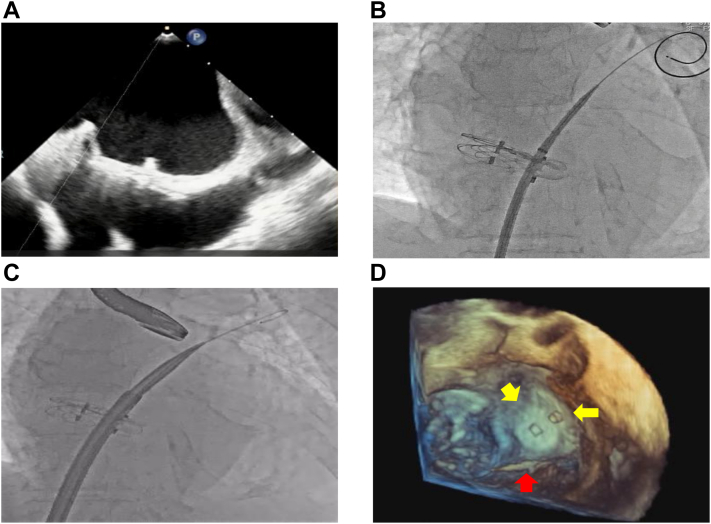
Images Demonstrating Transseptal Puncture and Device Passage (A) TEE bicaval view showing effective tenting of the inferoposterior interatrial septum with the SL-0 sheath. (B) Fluoroscopic image demonstrating successful passage of the SL-0 sheath across the interatrial septum following puncture. (C) Fluoroscopic image showing passage of the PASCAL Ace delivery system through the interatrial septum. (D) Three-dimensional TEE image showing the PASCAL Ace guiding sheath (red arrow) crossing the interatrial septum, with 2 previously implanted septal closure devices also visualized (yellow arrows). TEE = transesophageal echocardiography.
